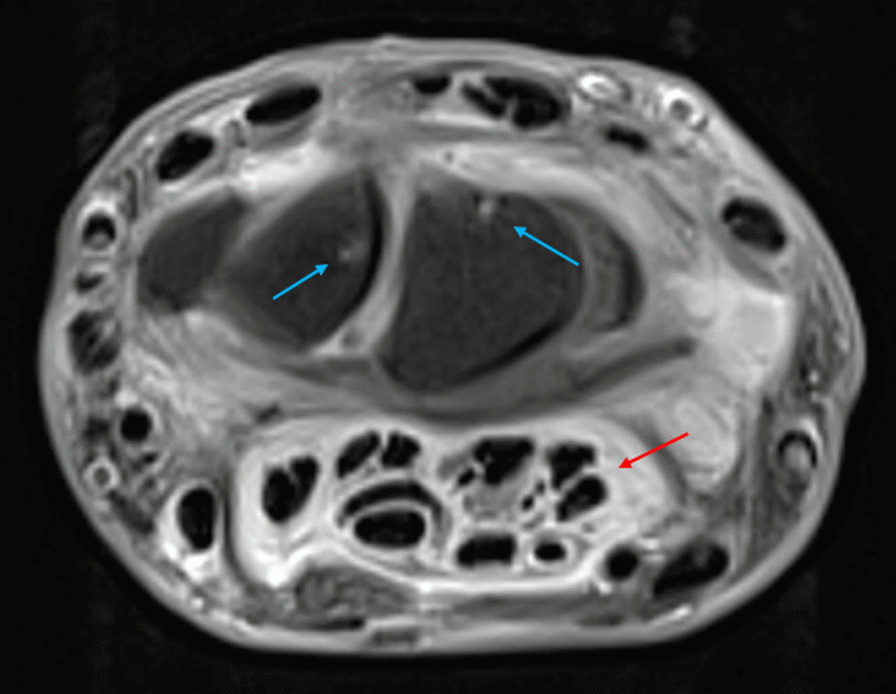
Magnetic resonance imaging of the right hand, transversal view: red arrow shows tenosynovitis of superficial and deep flexor tendons; blue arrows show carpal bones erosions
